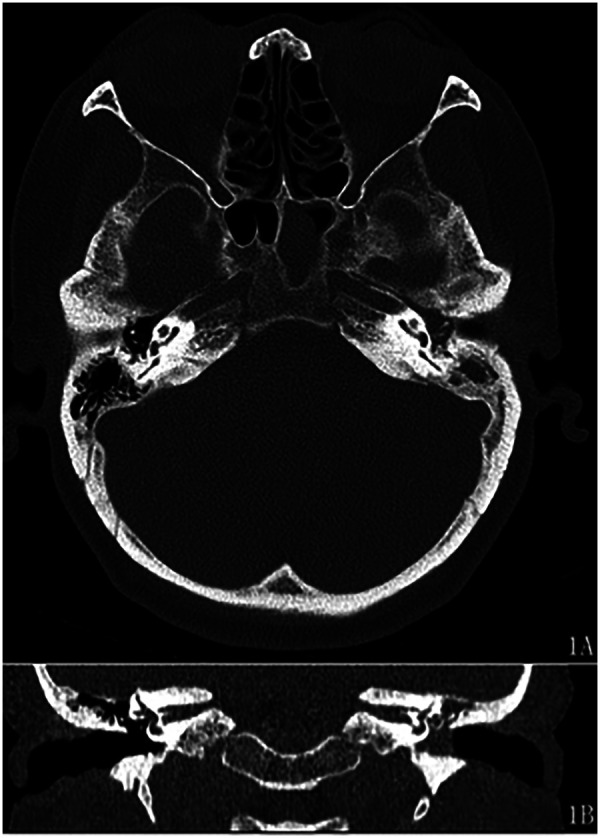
Temporal bone CT scans (axial and coronal views). Axial CT (1A) and coronal CT (1B): a soft tissue density mass occupying the left mastoid process, epitympanum, and mesotympanum.
